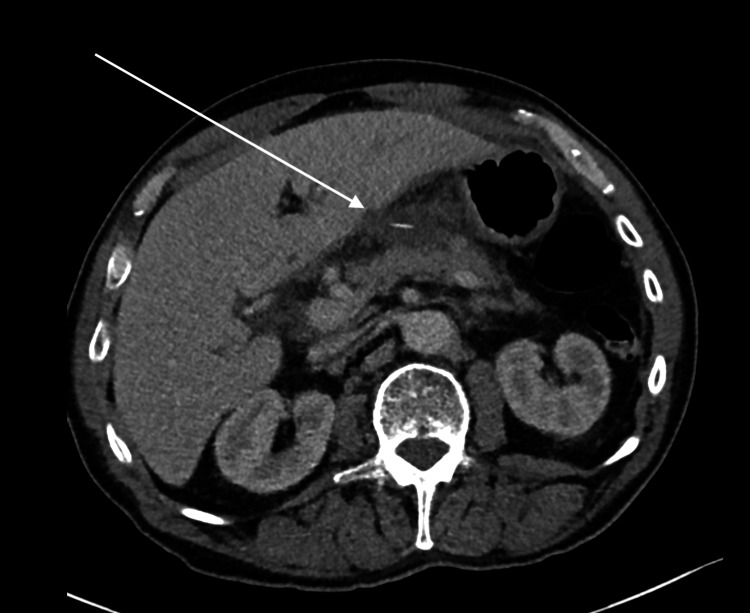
Computed tomography axial view of the upper abdomen. The arrow points to a hypodense collection within the lesser sac and a subcapsular hepatic collection measuring 25 × 38 × 33 mm (AP × TV × CC). At the center of this collection is a linear fish bone. AP, anteroposterior; TV, transverse; CC, craniocaudal
